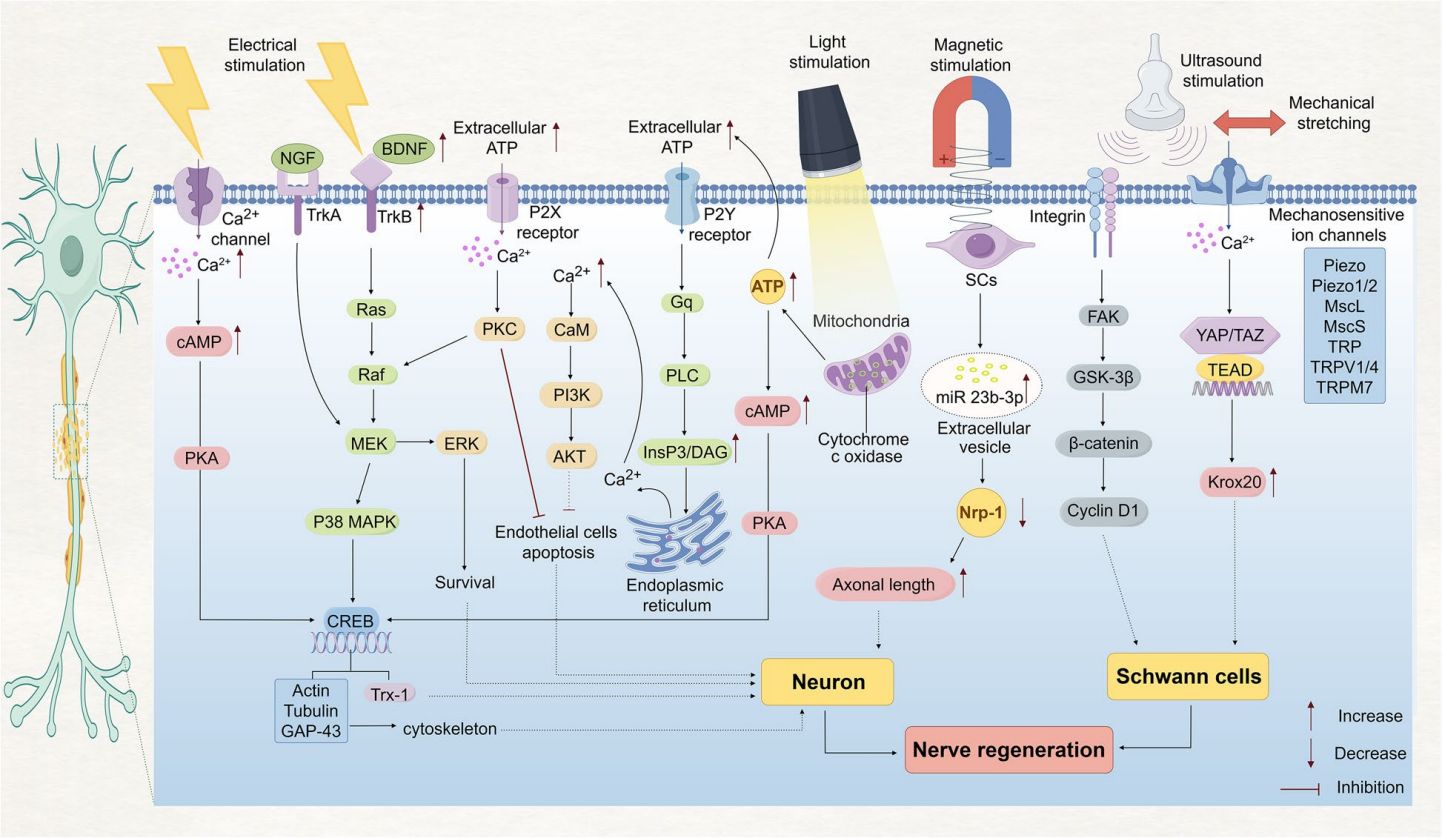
The mechanism of physical modulation to promote peripheral nerve regeneration. As shown, electrical stimulation, ultrasound, light, magnetic stimulus, and mechanical stretching can respectively employ various key molecules and signaling pathways as well as the production of energy substances to promote axonal growth, proliferation, and remyelination of Schwann cells after the peripheral nerve injury (By Figdraw)
